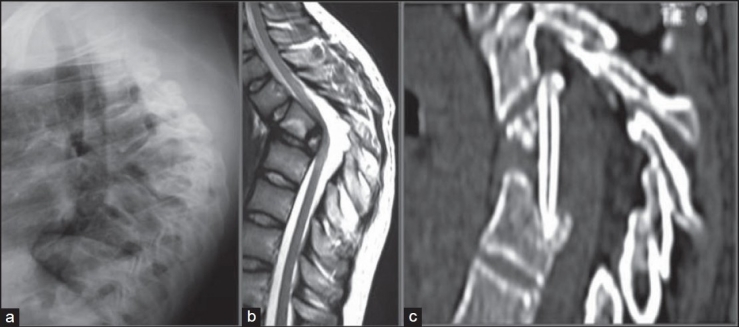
Lateral X-ray of dorsal spine (a) in a 20-year-old boy with late onset paraplegia: The patient had TB spine at the age of five years. D5–7 has become a bone block with 90° kyphosis and child has reported with mild motor weakness with spasticity. T2 WI mid sagittal MRI (b) scan shows kyphotic spine with internal salient indenting and atrophied spinal cord. The reconstructed mid saggital CT image (c) after internal kyphectomy: The picture shows spacious spinal cord with bridge tricortical graft *in situ*. The kyphus was not corrected and the patient showed significant neural improvement
